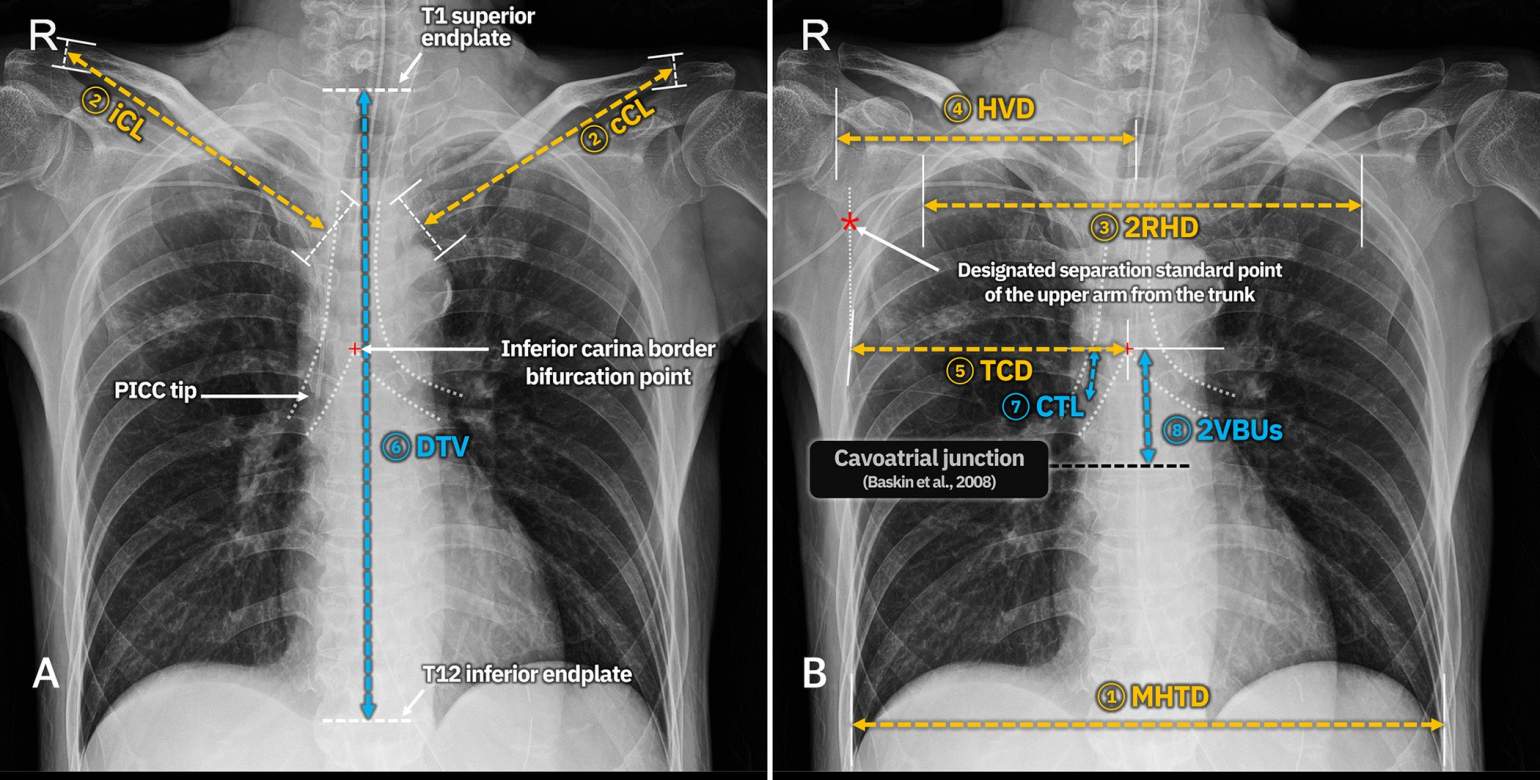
Radiologic parameters on anteroposterior chest radiographs. Horizontal elements are marked with yellow and vertical elements with blue. The parameters are as follows: MHTD, maximal horizontal thoracic diameter; iCL, ipsilateral clavicle length; cCL, contralateral clavicle length; 2RHD, second ribs horizontal distance; HVD, humero-vertebral distance; TCD, thoraco-carinal distance; DTV, distance of thoracic vertebrae; CTL, carina to catheter tip length; 2VBUs, vertical distance of two vertebral body units; T1, first thoracic vertebra; T12, twelfth thoracic vertebra; PICC, peripherally inserted central catheter.
